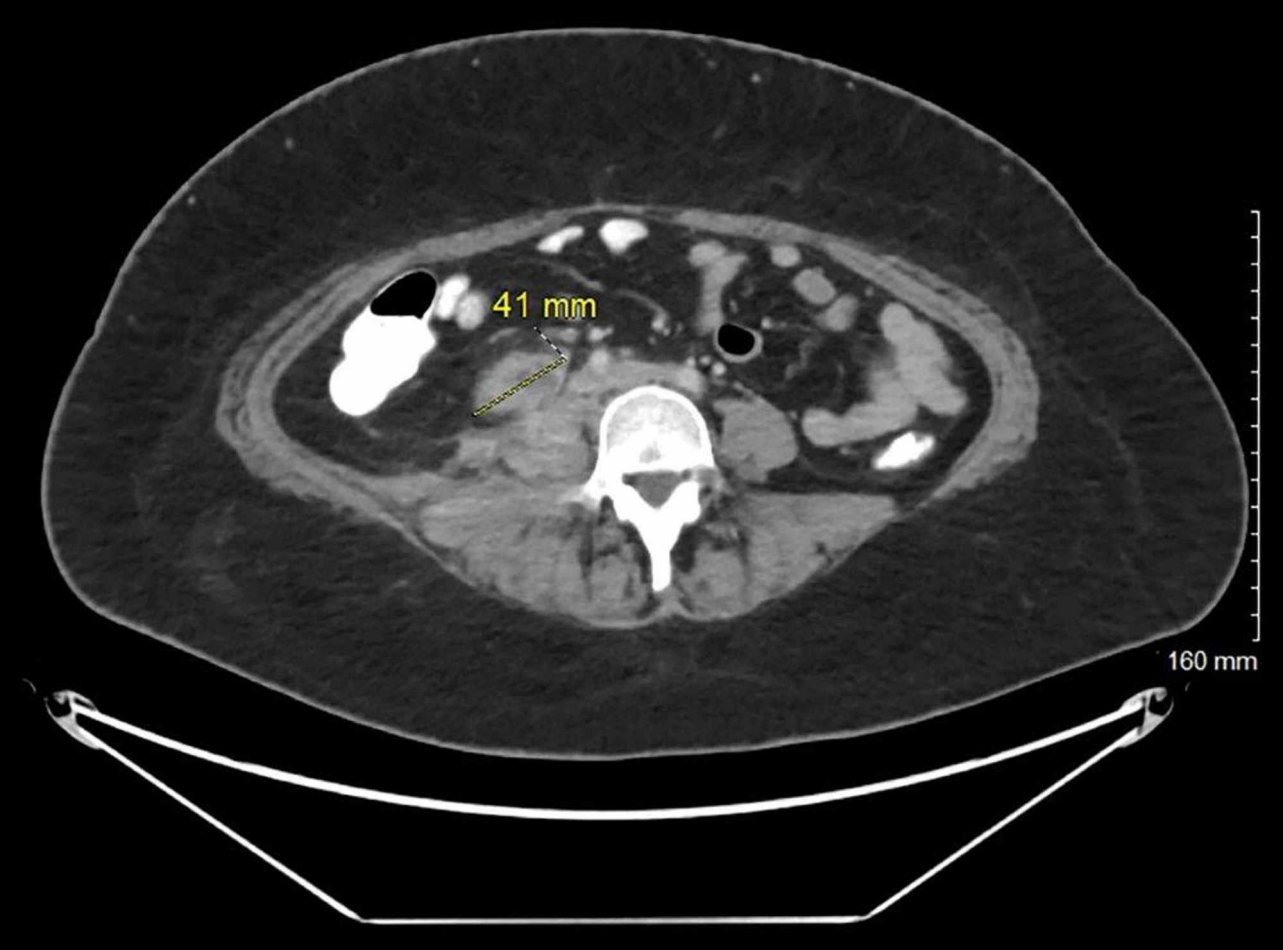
CT abdomen/pelvis with contrast at four weeks Complete resolution of the psoas abscess, a necrotic right retroperitoneal mass measuring approximately 4.1 cm, which is inseparable from the right iliopsoas muscle, which is consistent with metastatic disease CT: computed tomography
